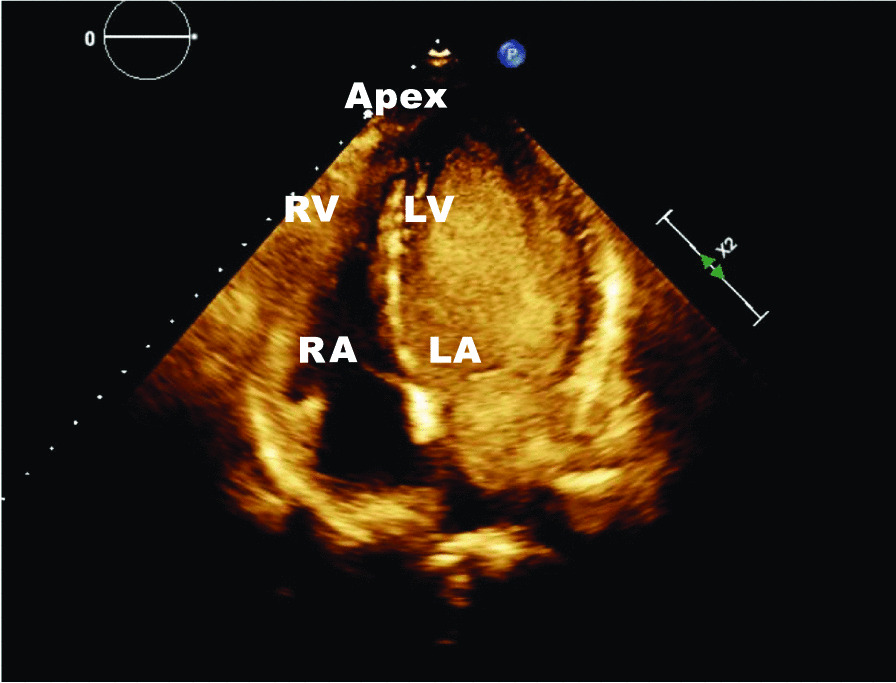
Apical four-chamber view shows solely an opacification of the left heart (*LV* left ventricle, *LA* left atrium) without contrast media affecting the right sided cavities (*RV* right ventricle, *RA* right atrium)
